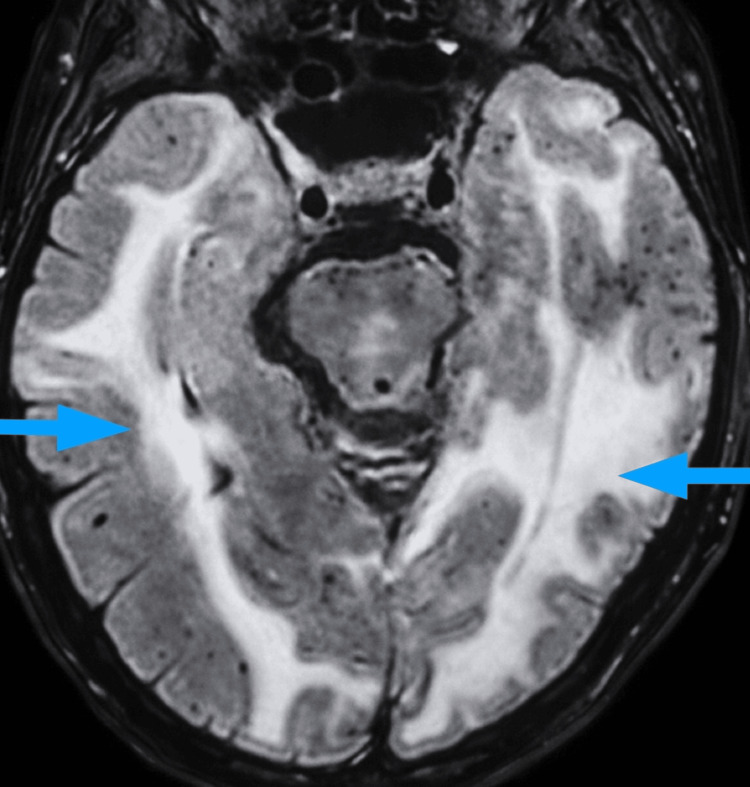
Axial fluid-attenuated inversion recovery (FLAIR) MRI showing asymmetric bilateral cortico-subcortical hyperintensities with posterior predominance, consistent with vasogenic edema (blue arrows).
